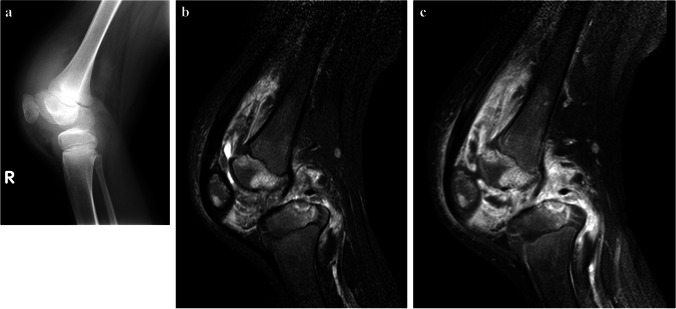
Images of the right knee in a 12-year-old boy with tuberculous tenosynovitis and osteomyelitis. There was a history of a swollen knee for 6 months, no history of trauma and although he was not septic, he was noted to be severely malnourished. a A lateral knee radiograph shows a large joint effusion, suspected synovial thickening and osteopaenia of the patella. No periosteal reaction or focal bone lesion is demonstrated. He proceeded to magnetic resonance imaging (MRI) following a failed attempt at joint aspiration. b A sagittal short tau inversion recovery MRI shows thickened low signal synovium lining the suprapatellar and popliteal fossa with a small amount of fluid in the suprapatellar space. There is also patchy oedema of the patella and femoral epiphysis with cartilage thinning and a focal erosive lesion of the tibial epiphysis. c A sagittal fat saturated T1 post-contrast MRI shows diffuse synovial and patchy multifocal bone enhancement. Synovial biopsy confirmed tuberculosis
